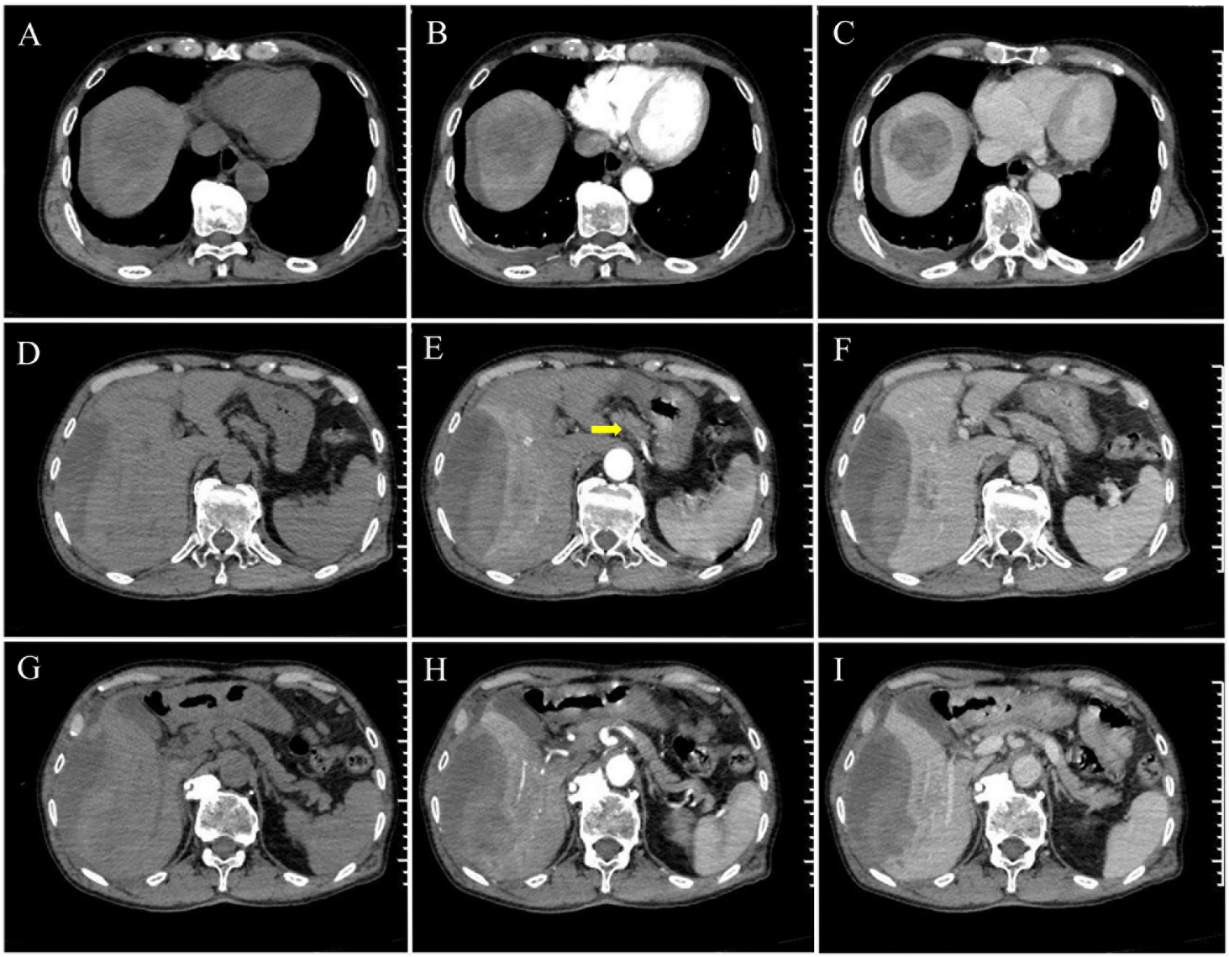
Contrast-enhanced CT images of the GHA patient. (A-I) The tumor is located in segments VI-VIII of the liver. (D-F) The gastric wall thickening and multiple lymph nodes in the gastric antrum (the yellow arrow). (D-I) The ruptured hepatic metastases with hemorrhage and perihepatic effusion.
